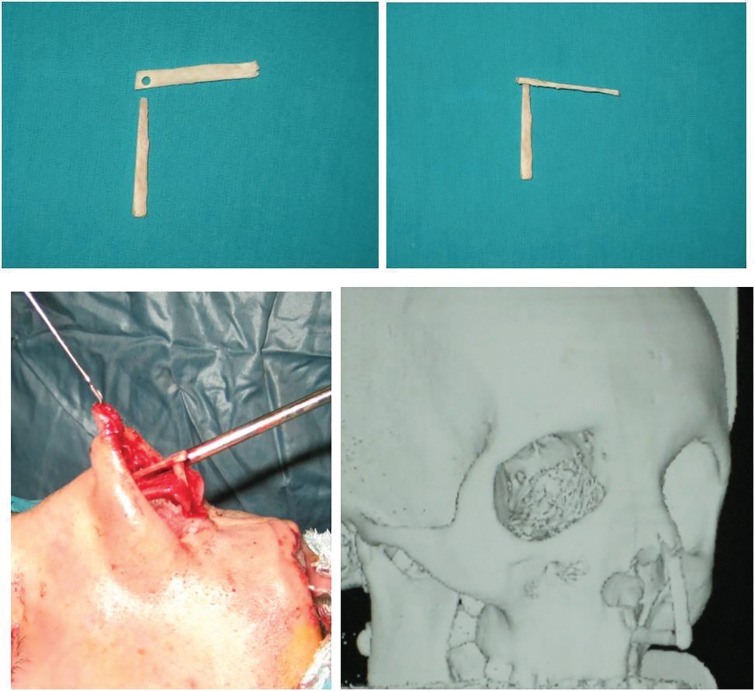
Two pieces of L-strut bone graft (above, left). A interlocked design (key in the keyhole pattern; above, right) L-strut bone graft was placed the nose (below, left). Three dimensional CT imaging of L-strut bone graft (below, right) is seen
